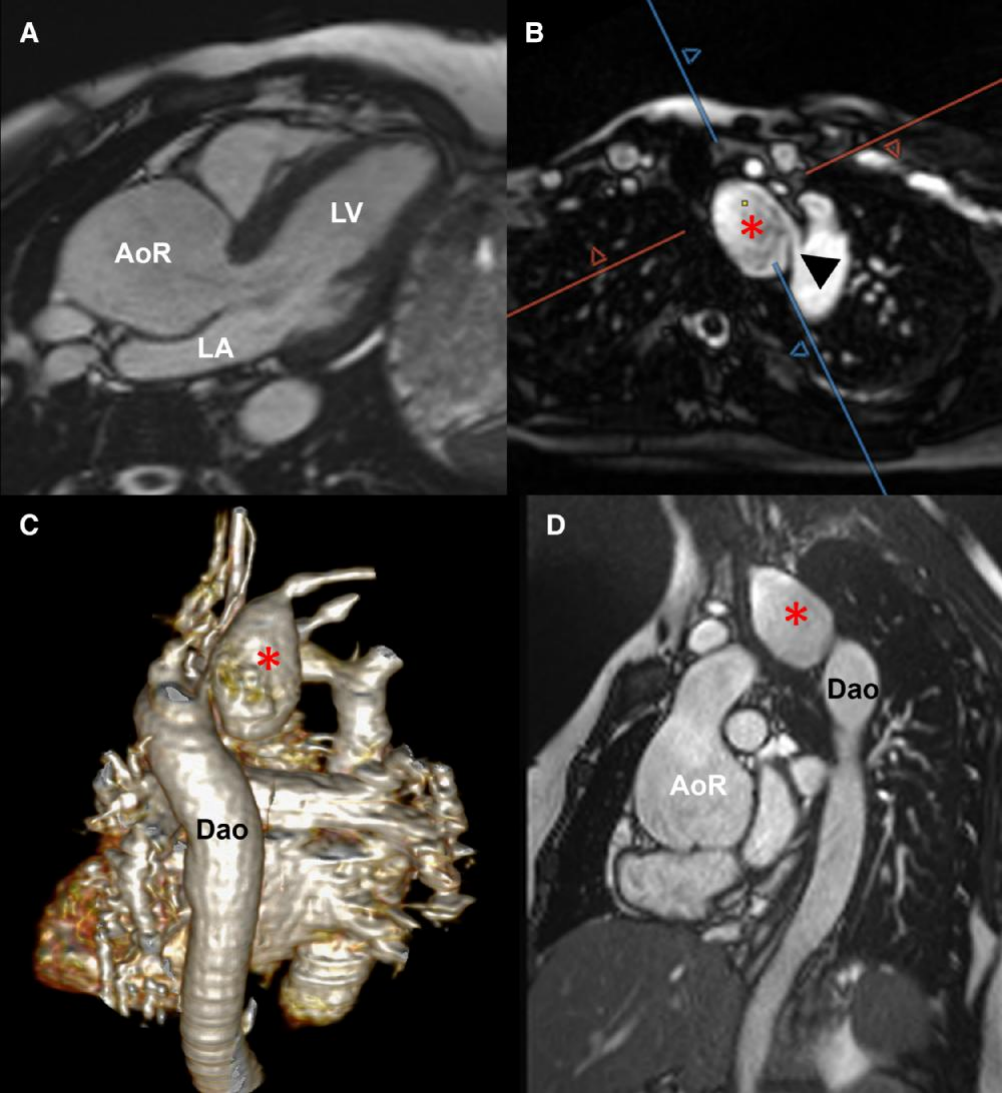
Cardiovascular magnetic resonance imaging without contrast at 21 weeks of gestation. Three-chamber view showing the enlarged aortic root (*A*). Quiescent-interval slice-selective (QISS) (*B*, *C*) and cine MRI (*D*) demonstrating the subclavian artery aneurysm (star) and the origin of the aberrant right subclavian artery (arrowhead). AoR, aortic root; Dao, descending aorta; LA, left atrium; LV, left ventricle; RV, right ventricle.
